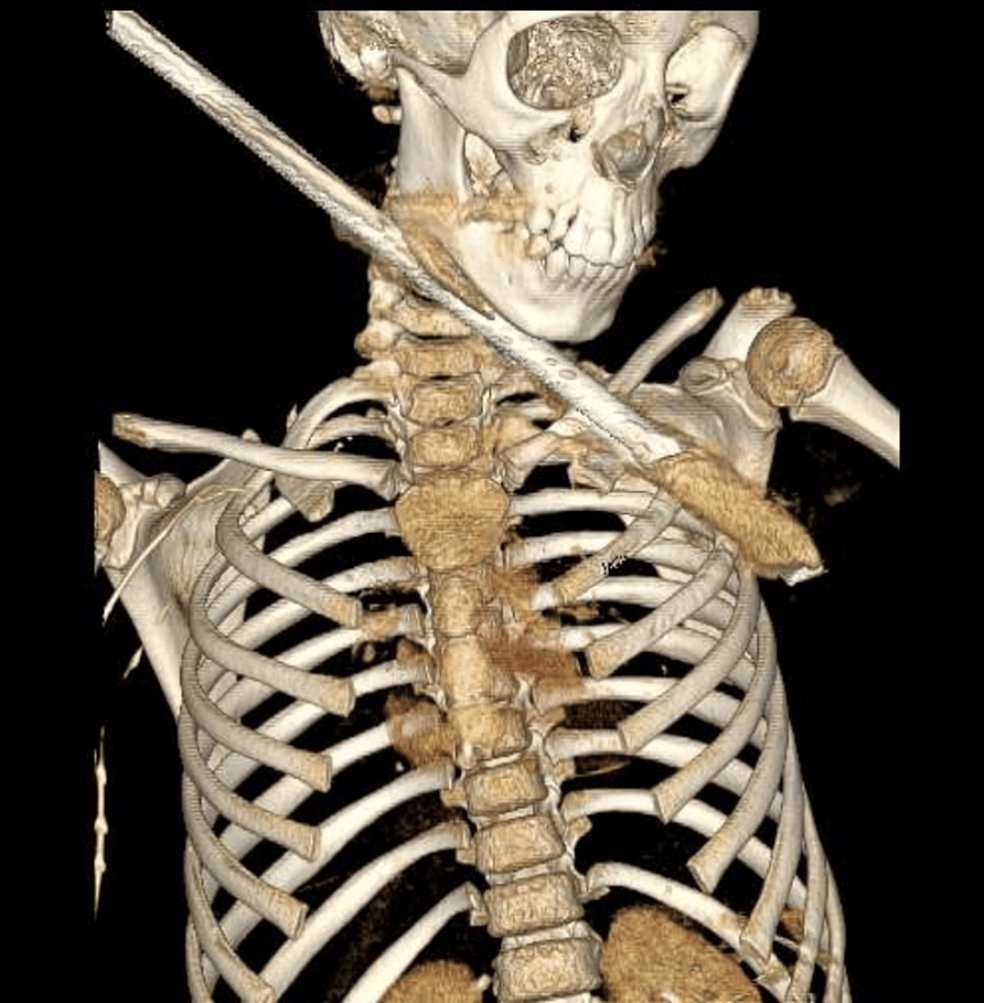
Three-dimensional visualization of the CT scan revealing a rod penetrating the left hemithorax through the middle part of the superior surface of the left clavicle and arriving at the axillary region.
